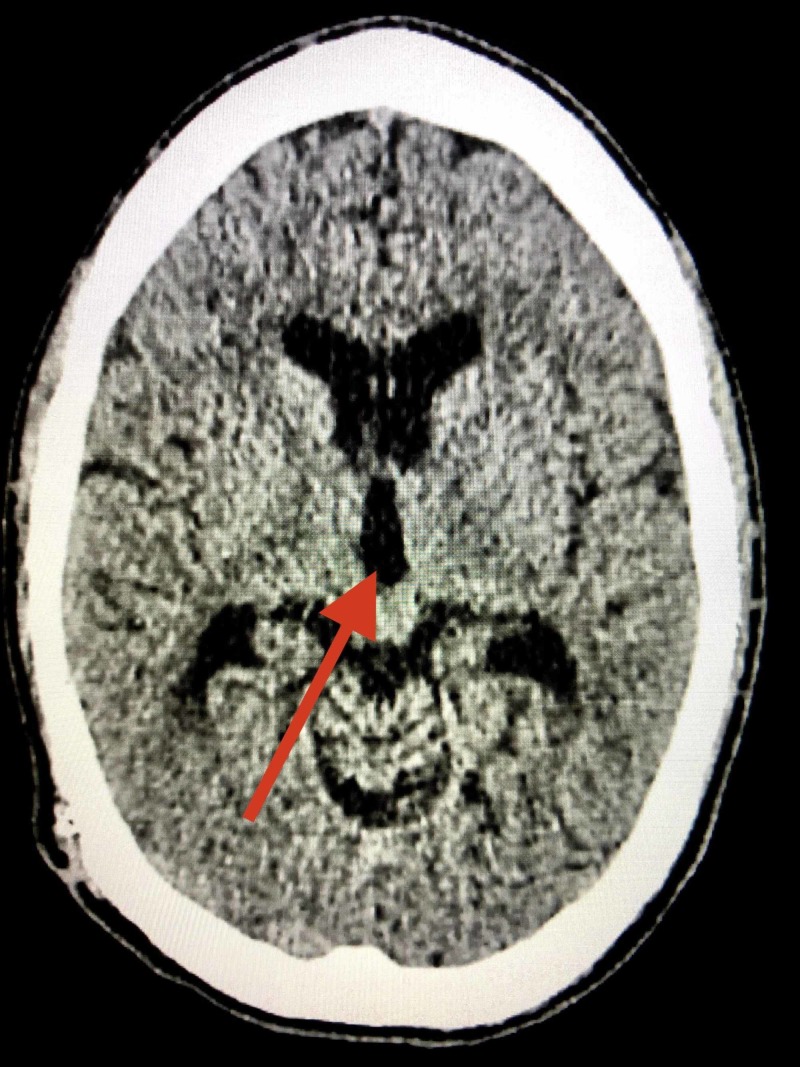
Post-operative axial computed tomography of the head demonstrating resolution of hydrocephalus; arrow indicating reduction in size of third ventricle
